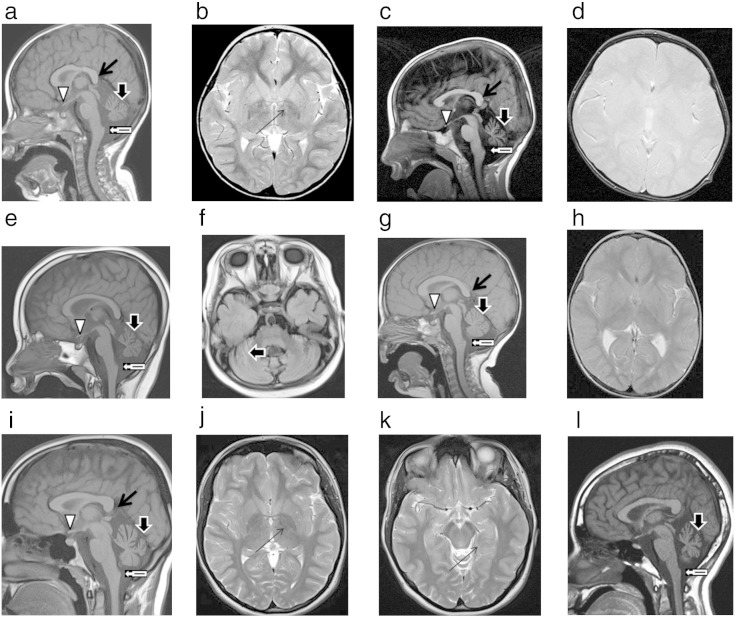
a. Midline sagittal T1 brain MRI of Case 1 at 27 months. b. Axial T2 weighted brain MRI of Case 1 aged 39 months, demonstrating iron deposition in the globus pallidus, which is absent on earlier imaging (a). c. Midline sagittal T1 brain MRI of Case 2 aged 33 months, demonstrating classical features of typical PLAN (symbol key and Table 3) and apparent claval hypertrophy. d. Axial T2* brain MRI of Case 2 aged 33 months, at the level of the globus pallidus; note the absence of iron deposition. e. Midline sagittal T1 brain MRI of Case 3 aged 4 years and 6 months, demonstrating classical features of typical PLAN and apparent claval hypertrophy. f. Axial FLAIR brain MRI of Case 3 aged 4 years and 6 months at level of cerebellum, showing high signal of the cerebellar cortex, indicative of gliosis. There is no iron deposition. g. Midline sagittal T1 brain MRI of Case 4 aged 22 months, demonstrating cerebellar atrophy, apparent claval hypertrophy and thin smooth splenium of the corpus callosum. h. Axial T2 weighted brain MRI of Case 4 aged 22 months, at the level of the globus pallidus. There is no iron deposition. i. Midline sagittal T1 brain MRI of Case 5 aged 11 years, demonstrating cerebellar atrophy and apparent claval hypertrophy. j. Axial T2 brain MRI of Case 5 aged 16 years demonstrating iron deposition in the globus pallidus. k. Axial T2 brain MRI of Case 5 aged 16 years demonstrating iron deposition in the substantia nigra. l. Midline sagittal T1 brain MRI of a 10 year old female with hemiplegic migraine and pathogenic *CACNA1A* mutation, demonstrating cerebellar atrophy and apparent claval hypertrophy. Table t0020:
